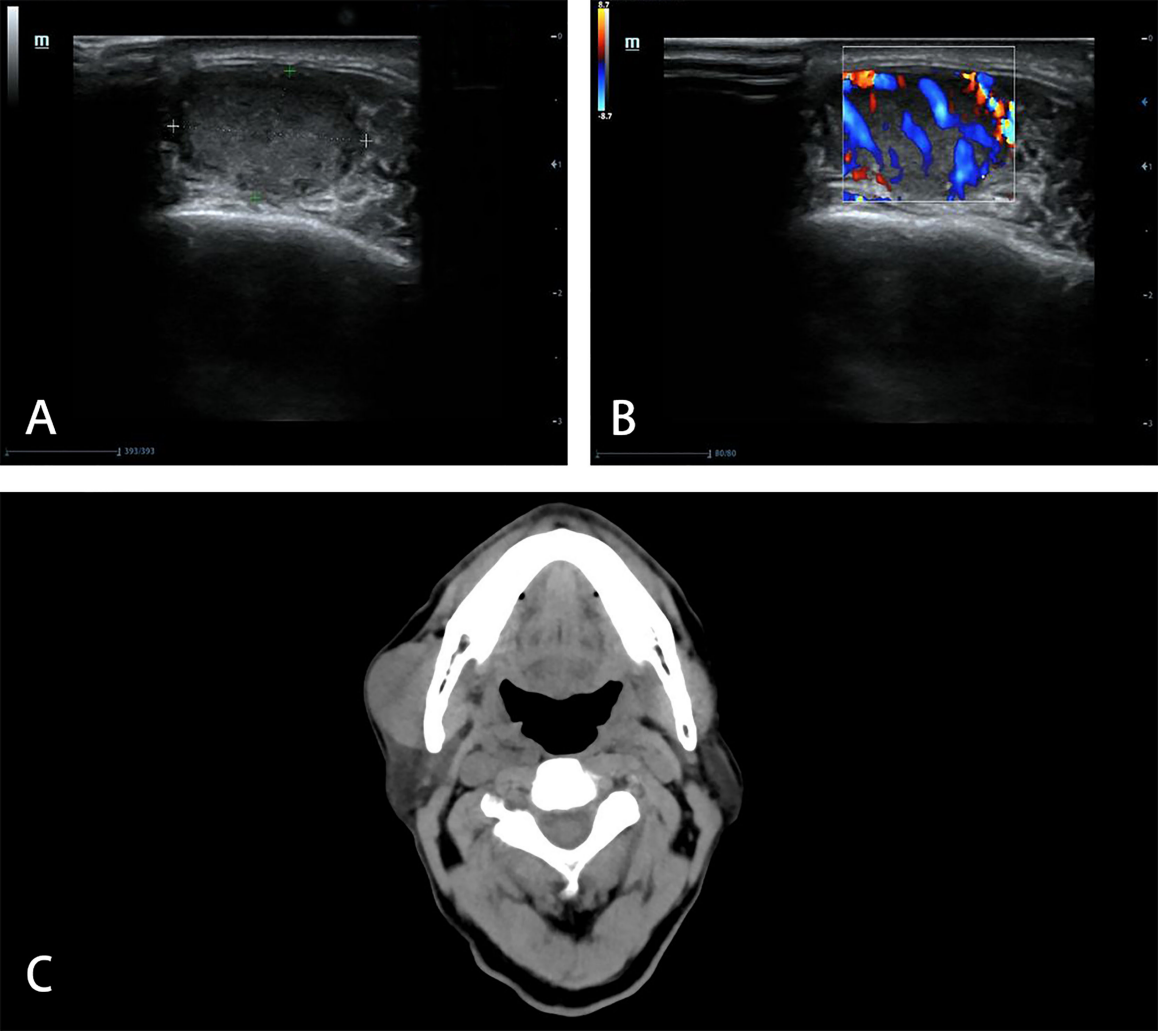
Imaging manifestations of masseter muscle metastasis in US and CT. US shows a hypoechoic (A), hypervascular (B) mass in the muscle layer of the masseter area in 2019. CT shows unclear boundary between the mass and right masseter muscle in 2021 (C).
